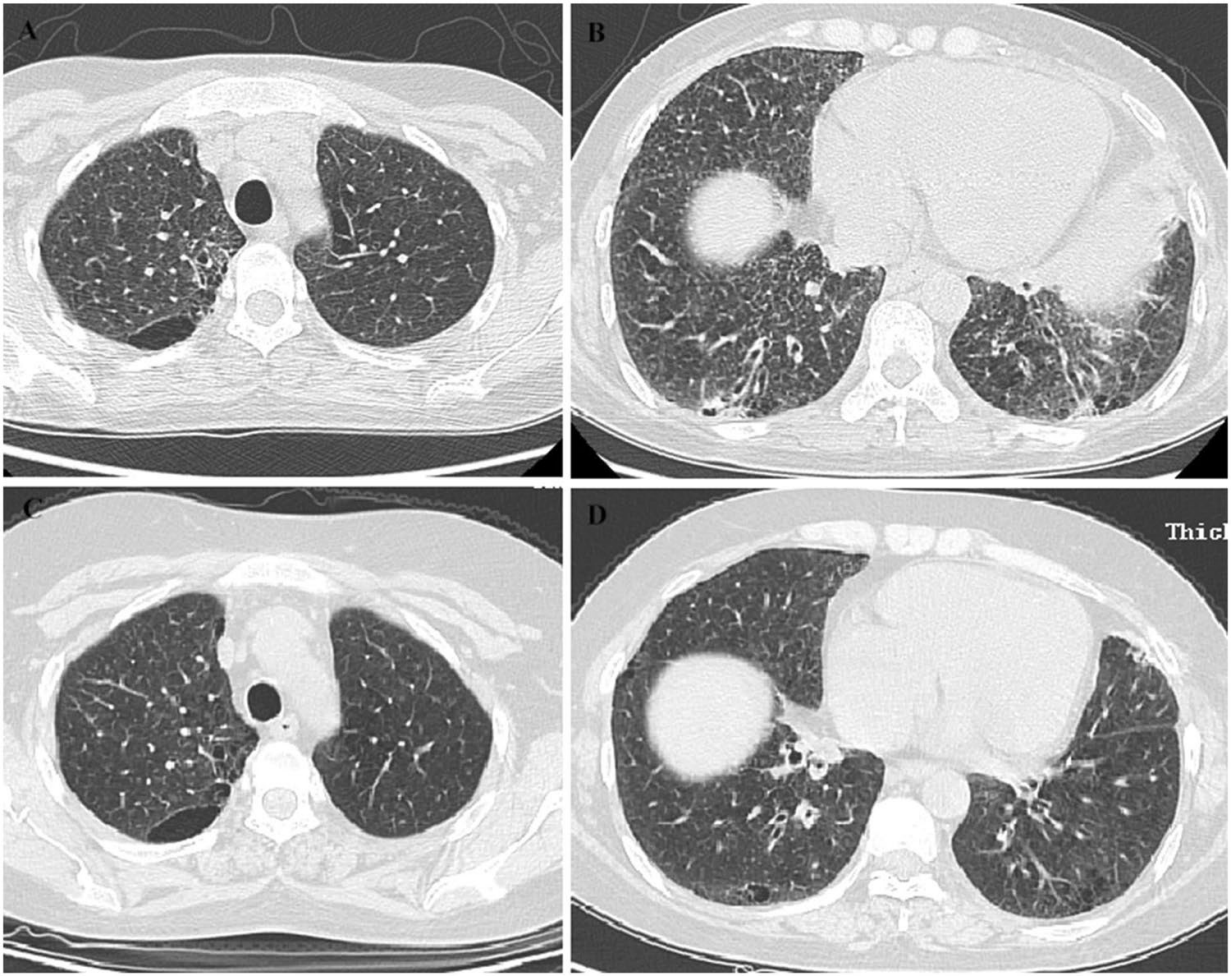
Case 2. (A,B) Chest CT (Jun 23, 2011) showed centrilobular nodules, patchy consolidation and GGOs, subpleural cysts and bronchiolectasis in both lower lobes. (C,D) Chest HRCT (Apr 18, 2012) demonstrated that centrilobular nodules, patchy consolidation and GGOs were all absorbed, and the changes of imaging were better than ten months before.
